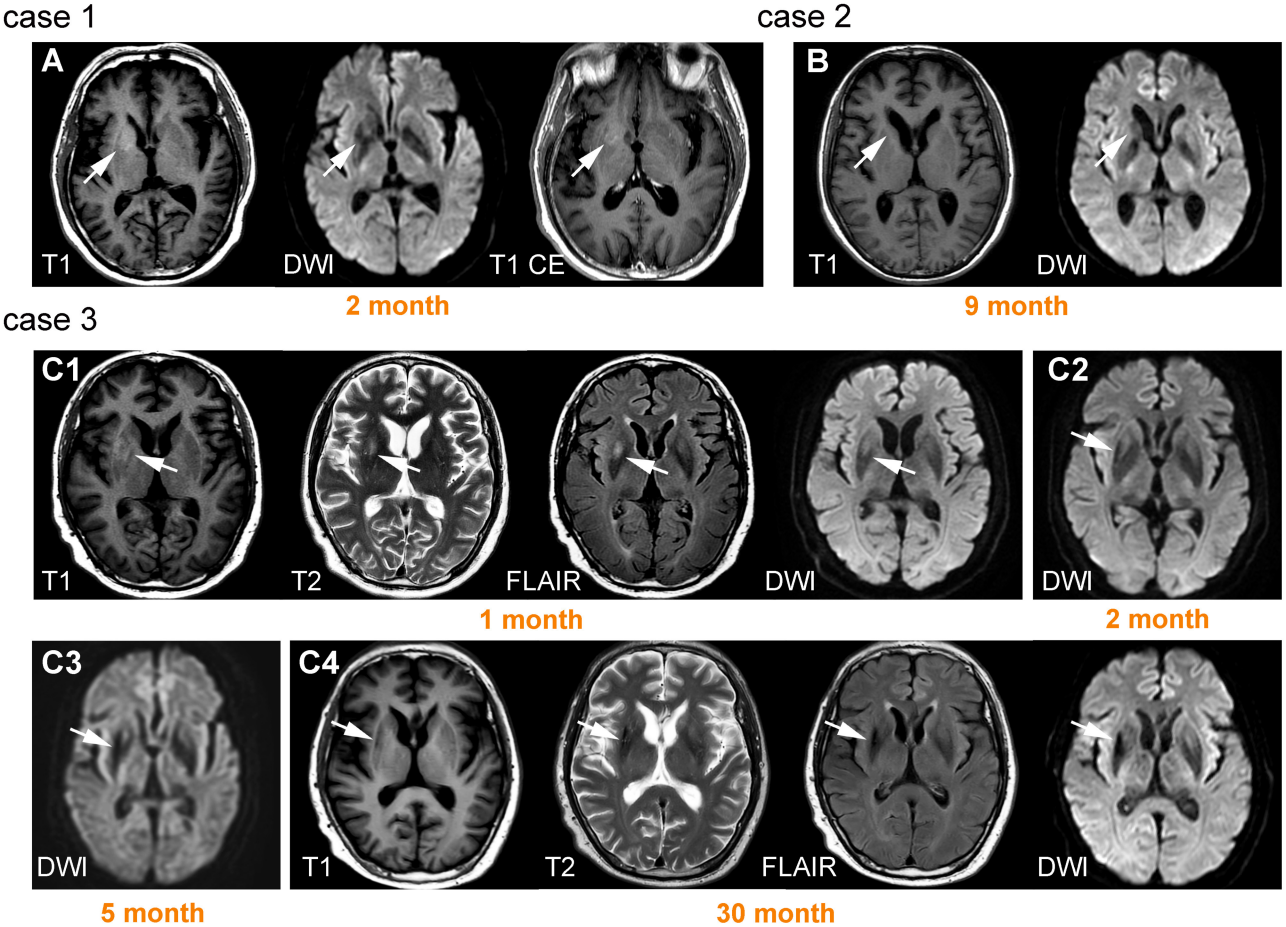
Lesions on basal ganglia and their signal change over time. Case 1 was a 66-year-old male patient with left faciobrachial dystonic seizure (FBDS). A lesion was observed on the right basal ganglia (BG) with T1 hyperintensity and diffusion-weighted imaging (DWI) hypointensity, accompanied by ring-shaped enhancement (A). Case 2 was a 57-year-old male patient with epileptic seizure. Brain MRI showed prominent right caudate nucleus atrophy and DWI hypointensity 9 months after onset (B). Case 3 was a 61-year-old male patient presenting with left FBDS. Hyperintensity on T1, T2, fluid-attenuated inversion recovery (FLAIR), and DWI were initially observed on right BG (C1). Hypointensity on DWI began to appear 2 months after onset (C2) and became more prominent accompanied by atrophy 3 months later (C3). High signals were replaced by hypointensity 30 months after onset (C4).
